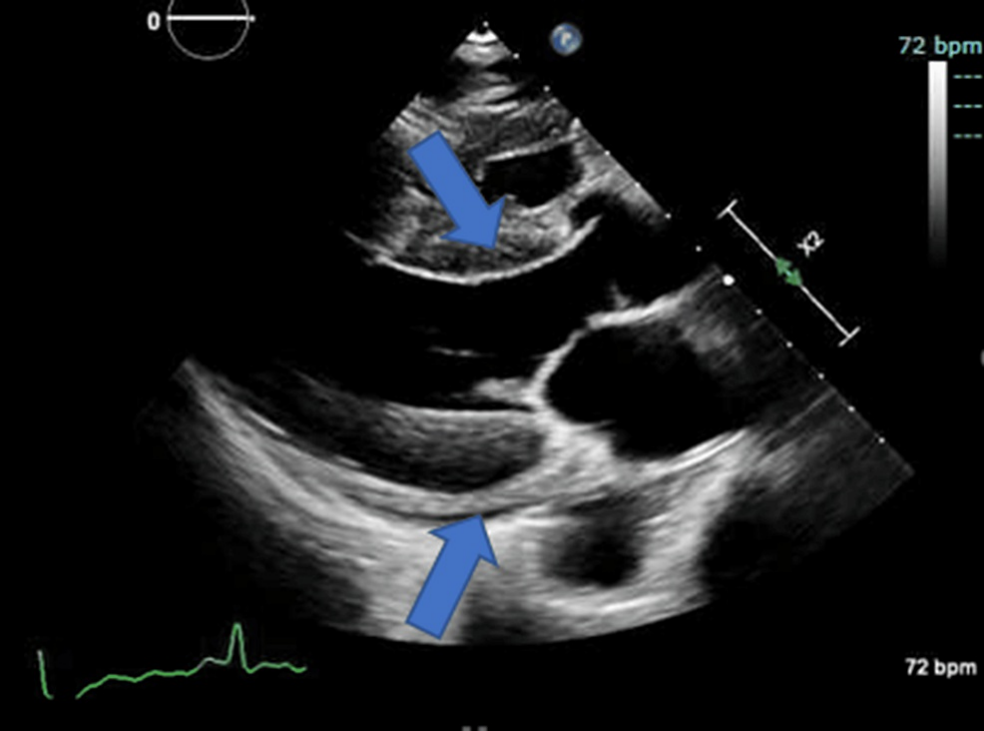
Echocardiogram showing mild global hypokinesis of the left ventricle, mild concentric left ventricular hypertrophy, and hyperechoic myocardium with no major valve insufficiencies.
